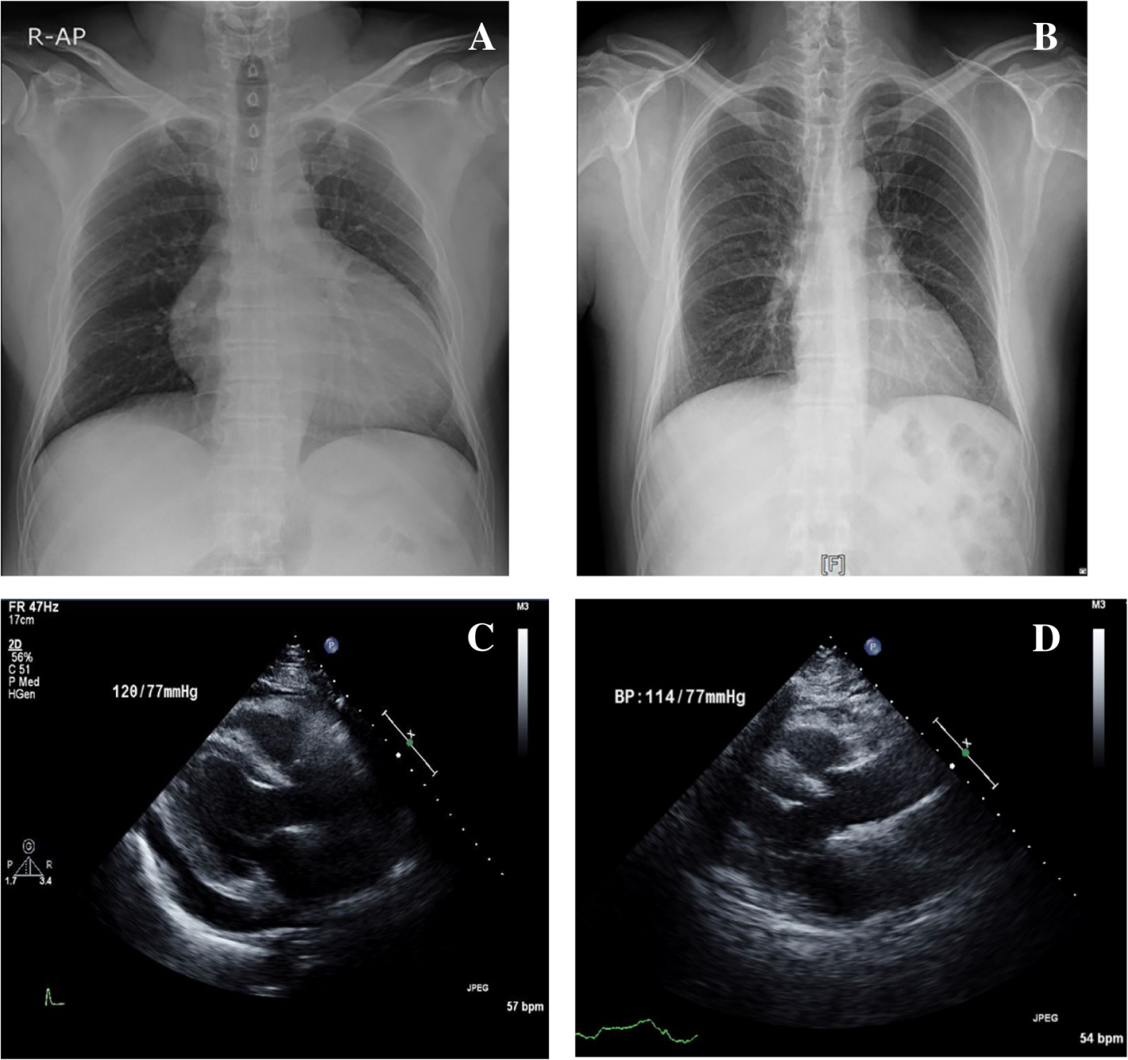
Image findings of the patient. Chest radiography shows cardiomegaly without pleural effusion at initial admission (a) and decreased cardiomegaly after discharge (b). Transthoracic echocardiography shows moderate pericardial effusion at initial admission (c) and completely recovered pericardial effusion at one-year later (d)
